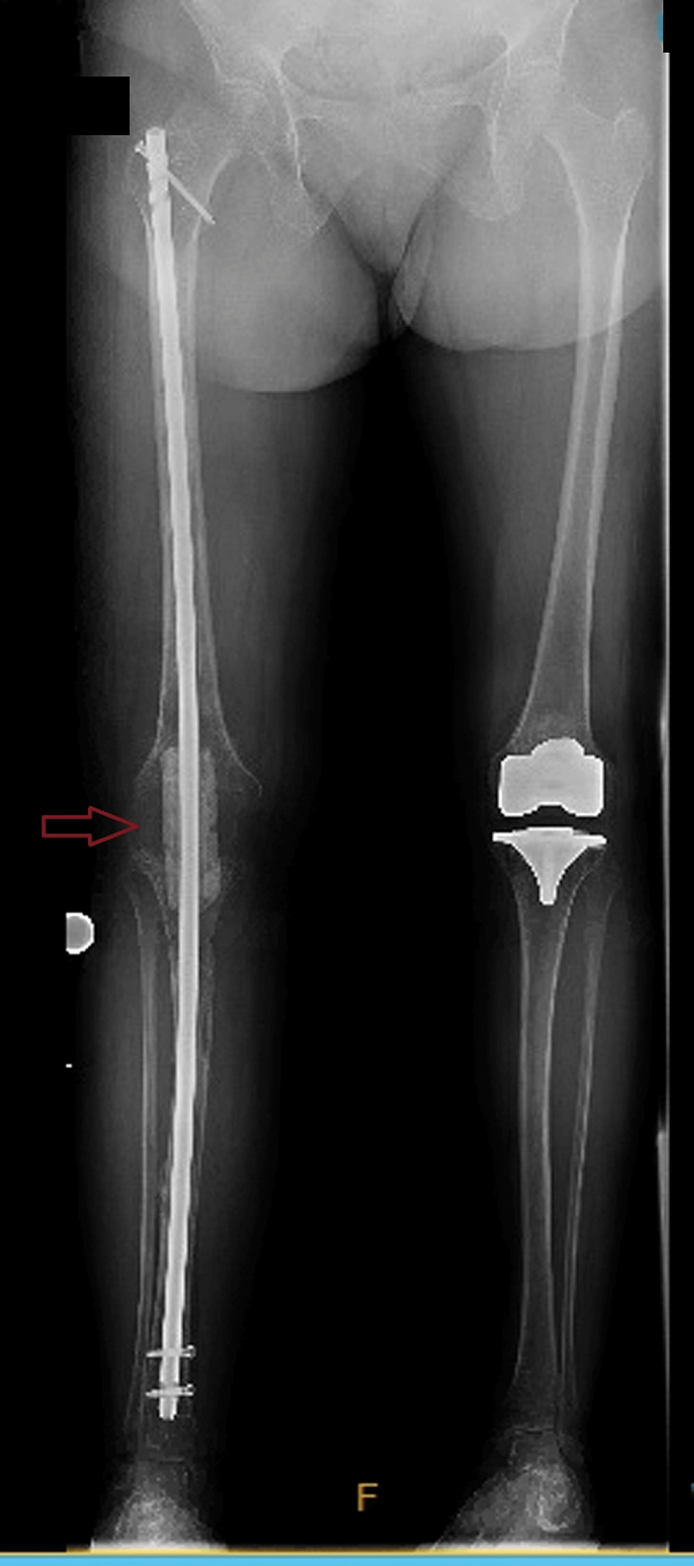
Long nail Anteroposterior plain radiograph showing the right knee long antibiotic-cement-coated intramedullary nail with the Palacos bone cement spacer block (arrow)
